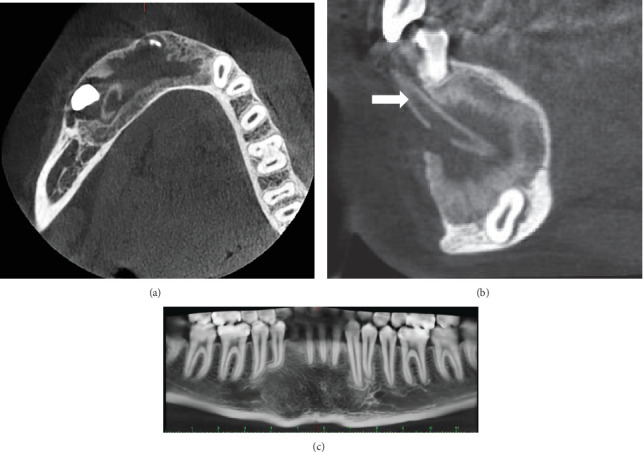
(a) CBCT axial section: 1-year follow-up (2015). Formation of trabecular bone at the lesion periphery and significant reduction in size are observed. (b) CBCT sagittal section: 1-year follow-up (2015). Peripheral new bone formation with reduced vestibule-lingual bulging is evident. The arrow indicates the cannula system. (c) CBCT panoramic view: 9-year postsurgical intervention (2023). Formation of bone tissue without alteration. Maintained anatomical structures and no signs of recurrence.
